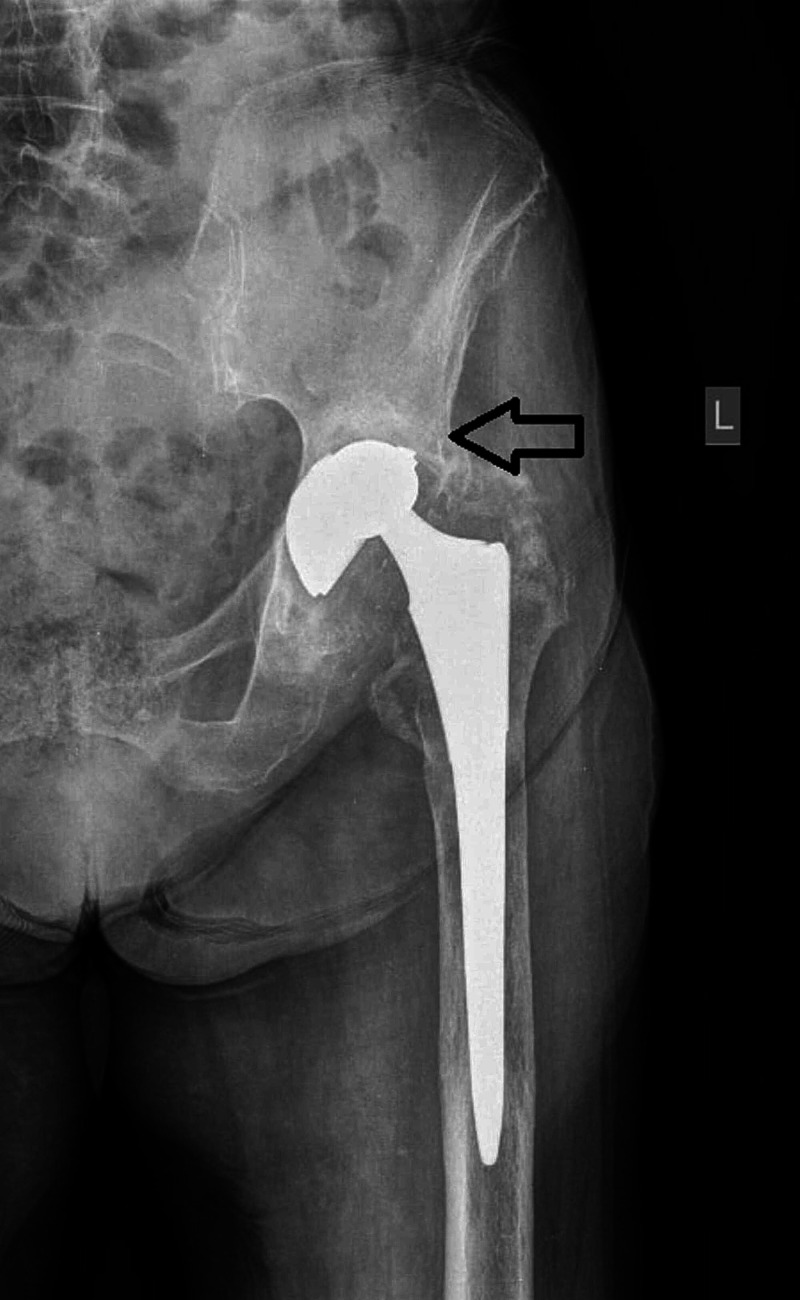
Anteroposterior X-ray of left hip arthroplasty on admission The black arrow shows the “unusual” dislocation of the joint.
